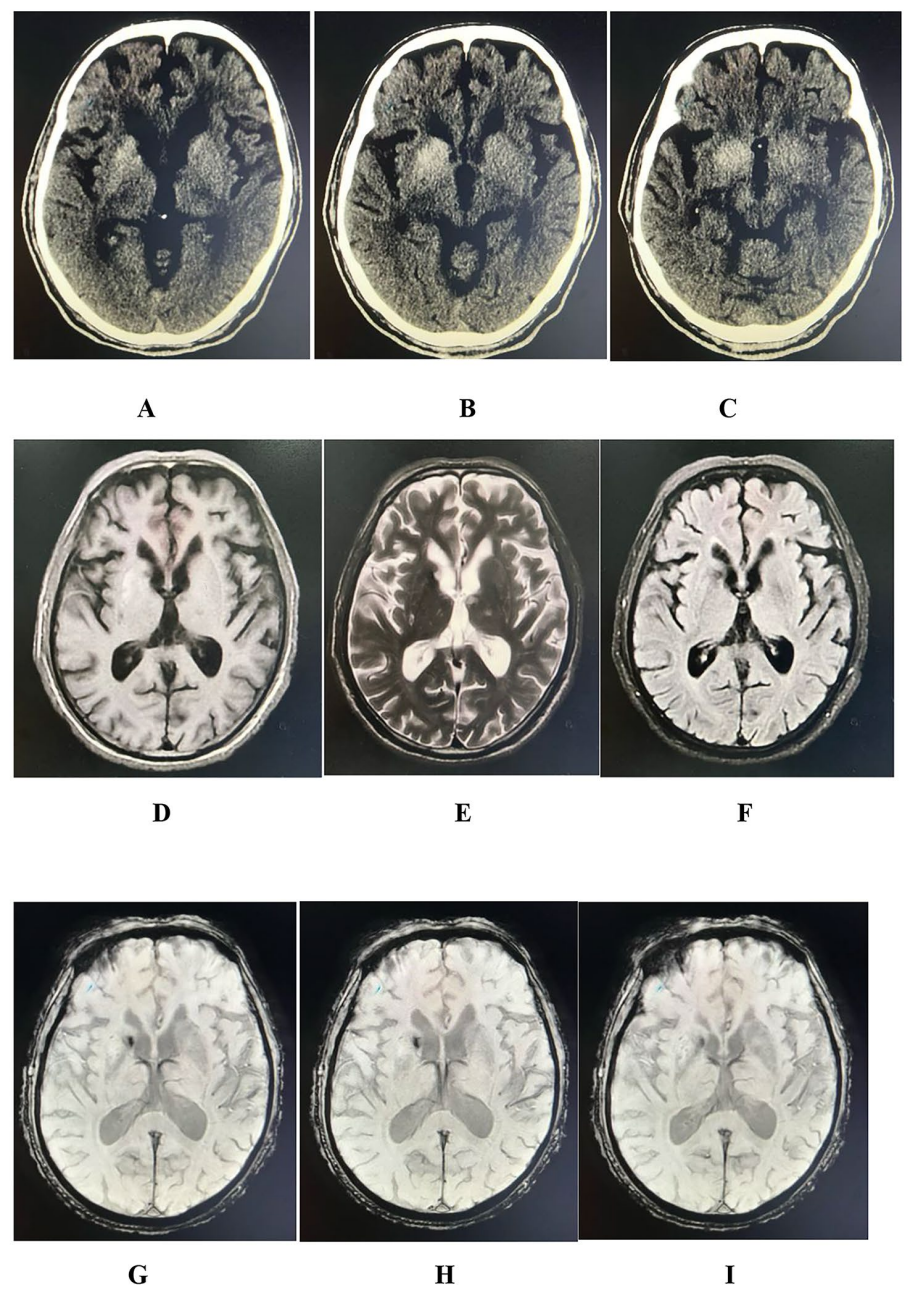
The patient’s imaging results. (A-C) Brain CT showed high density lesions in both basal ganglia regions, with more severe lesions on the right. (D-F) Brain MRI shows abnormal signals at the right head of caudate nucleus, with T1 showing high signal, T2 and Flair showing low signal. (G-H) SWI shows low signal at the head of the right caudate nucleus
